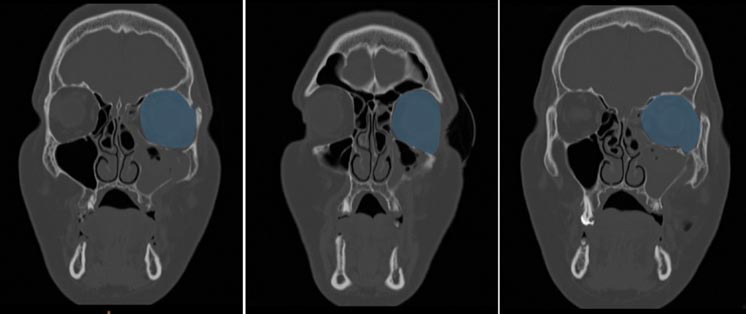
Preoperative computed tomography (CT) views of the traumatized left orbit in the coronal plane
